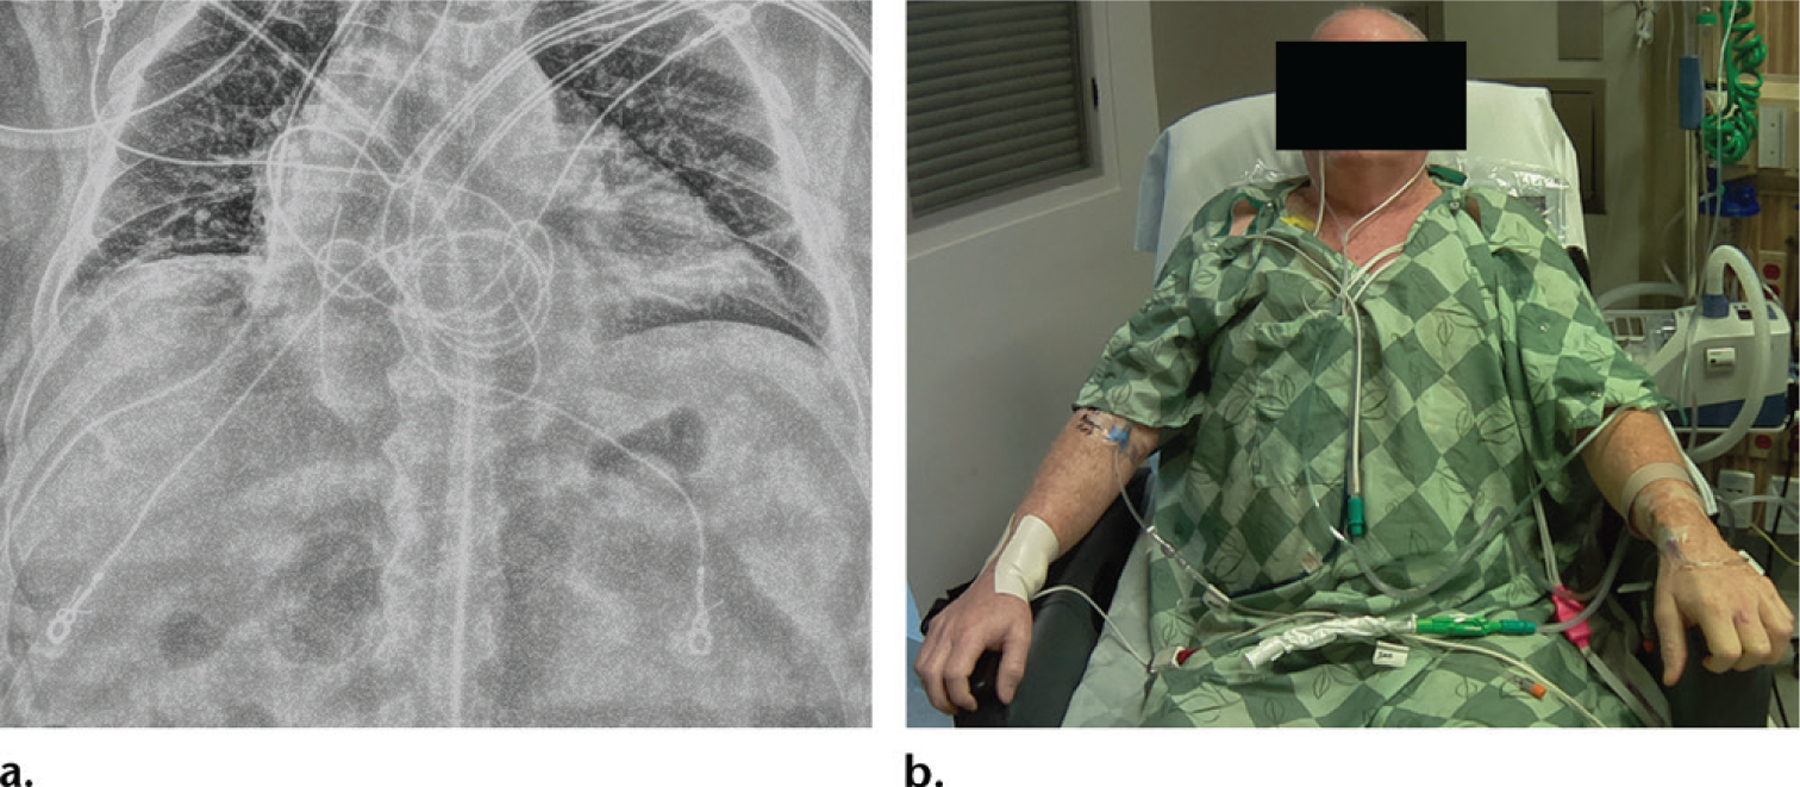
(a) Frontal abdominal radiograph obtained to exclude a diagnosis of pneumoperitoneum shows no free air. However, it was unclear from the radiograph whether the image was obtained with the patient in a supine or upright position, as the radiopaque marker was missing. (b) Photograph shows the patient in a near-erect position, adding confidence to the impression that there is no free air on the radiograph.
